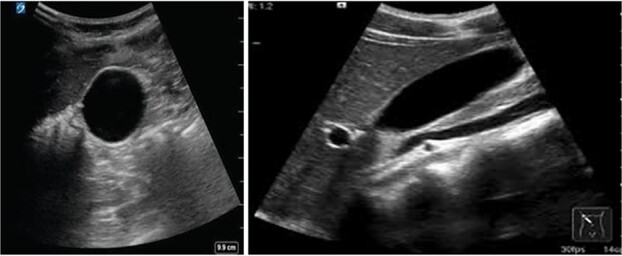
Normal gallbladder ultrasound after the drug discontinuation.
